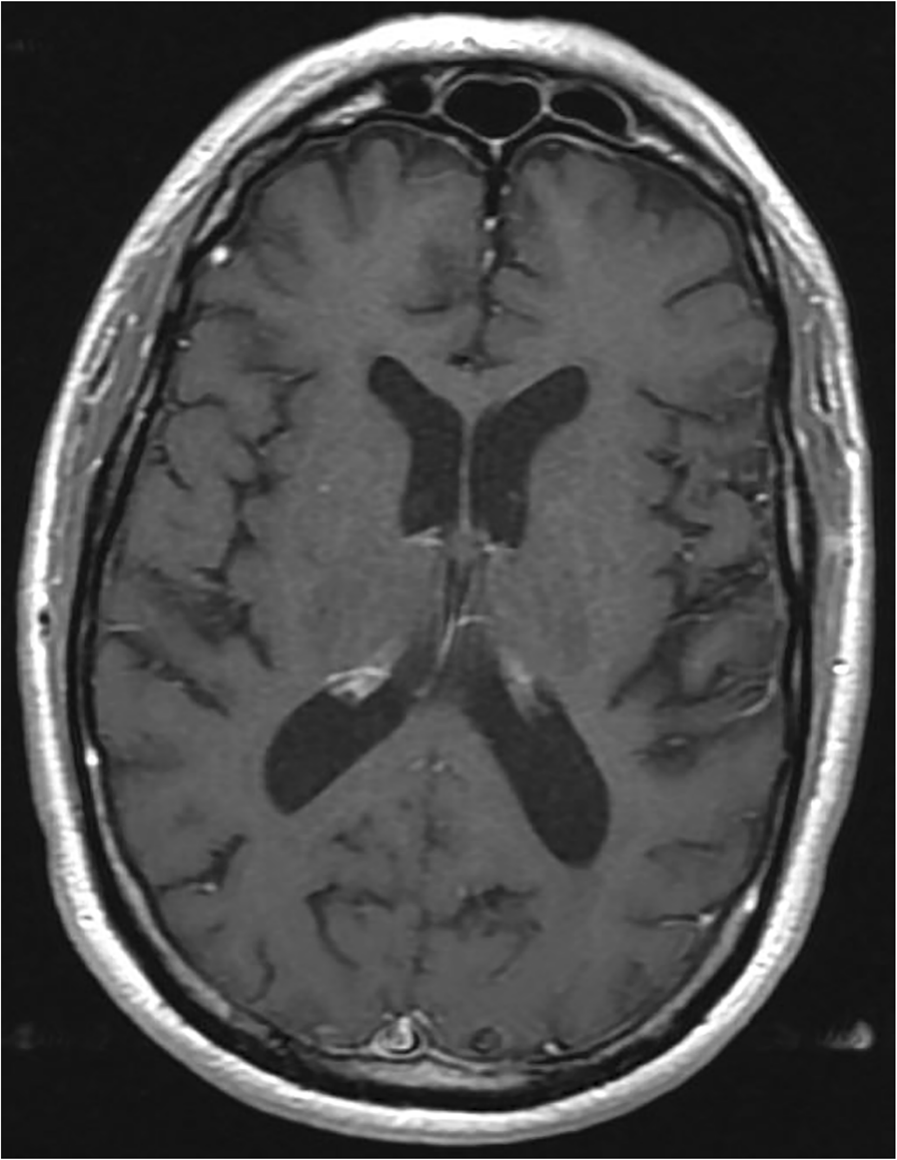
Brain MRI obtained 4 months after starting methotrexate in setting of neurological improvement. T1-weighted contrast-enhanced axial image through the parieto-occipital region shows resolution of leptomeningeal enhancement
